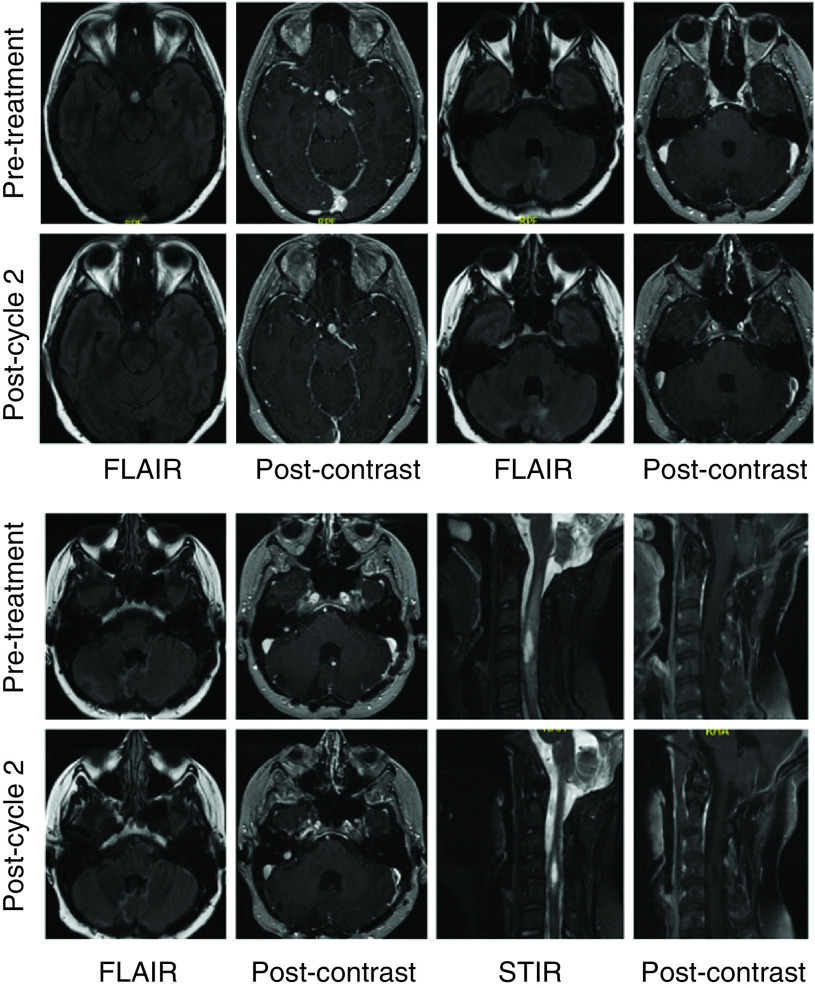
Pre- and post-treatment MRI brain and cervical spine for patient 1. Interval MRI of the brain and cervical spine performed with and without intravenous gadolinium contrast reveals a decrease in the degree of enhancement of intracranial lesions (pituitary area, multiple posterior fossa lesions, cervical lesion) and reduced perilesional FLAIR and STIR signal intensity. T1 postcontrast images of the cervical spine are fat suppressed. FLAIR: Fluid-attenuated inversion recovery; STIR: Short-TI inversion recovery.
